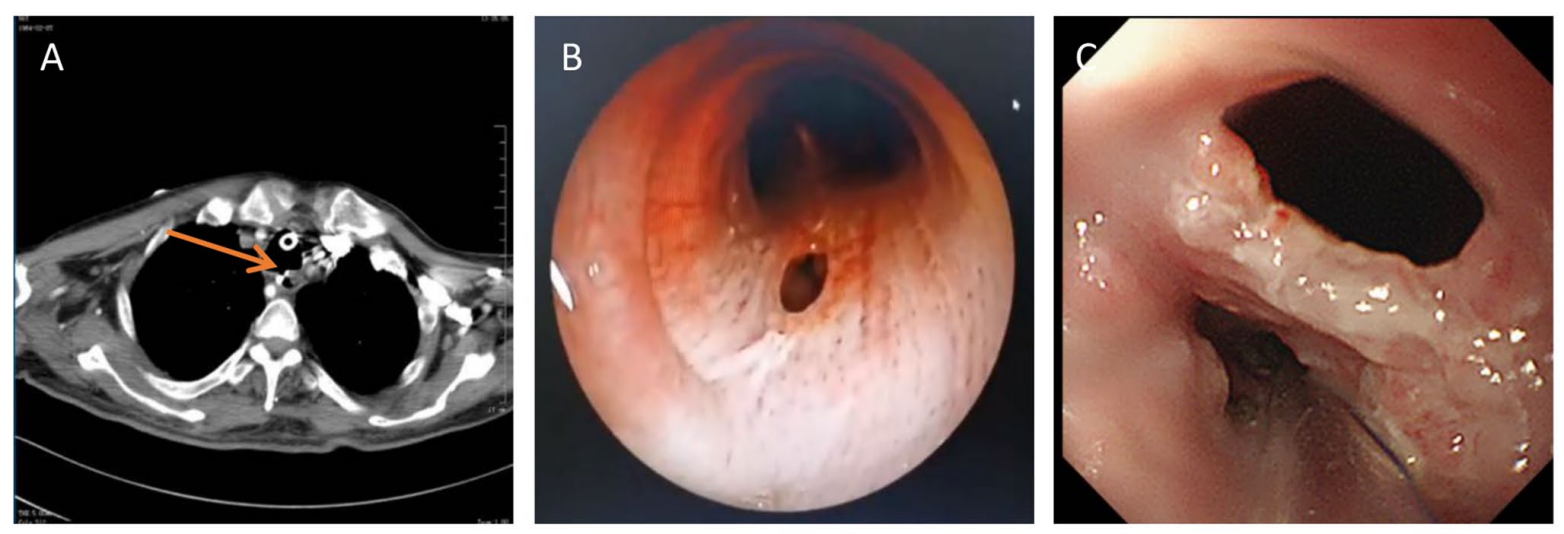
Diagnosis of TEF. (A) Diagnosis of TEF with chest CT ; (B) Confirmation of TEF location and size with bronchoscopy on tracheal side; (C) Confirmation of TEF location, size and cause with Digestive endoscopy on esophageal side
